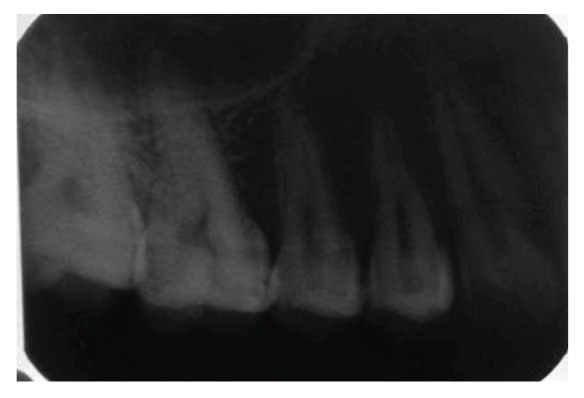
Intraoral periapical radiograph with 16 at 2-year followup.
